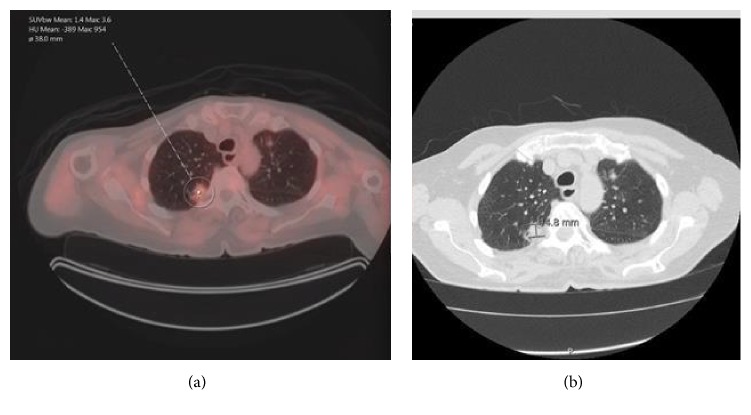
(a) On the left of the picture and (b) on the right of the picture. (a) PET CT showing increased uptake in the nodule. (b) CT Chest without contrast: 14.8 mm pleural-based mass-like density in the posterior medial right upper lobe.
